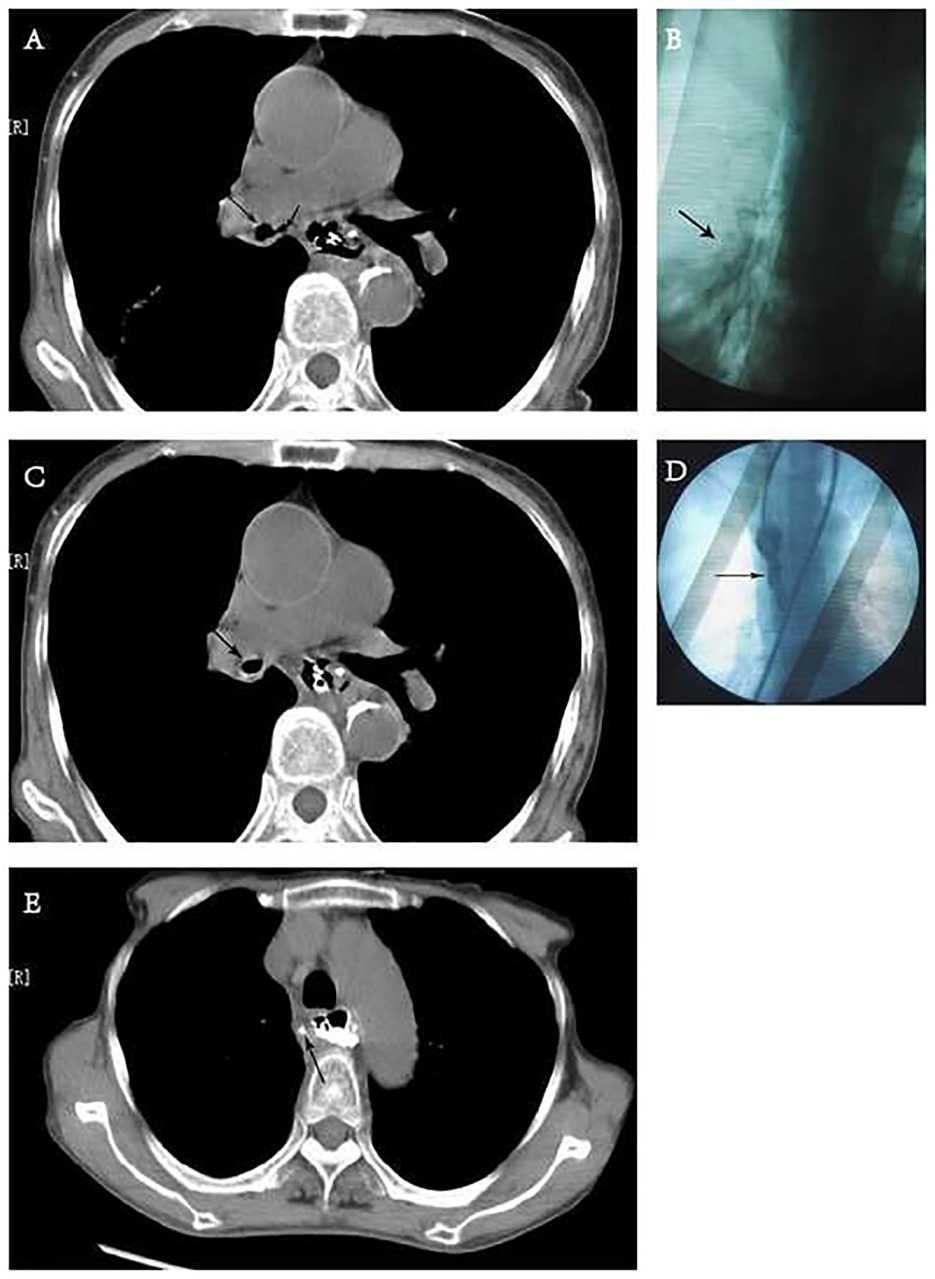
Chest computed tomography (CT) and fluoroscopy. (A) CT showed the bronchus communicate with mediastinal abscess. Long arrow: right inferior bronchus. Short arrow: mediastinum. (B) Dimeglumine flowed into right low lobe *via* mediastinal drainage tube through right inferior bronchus in chest fluoroscopy. Arrow: dimeglumine. (C,D) CT and fluoroscopy (pulled dimeglumine *via* mediastinal drainage tube) showed the fistula of bronchus is healed. (E) CT showed mediastinal abscess was healed after oral dimeglumine. Arrow: sinus tract after removing mediastinal drainage tube.
